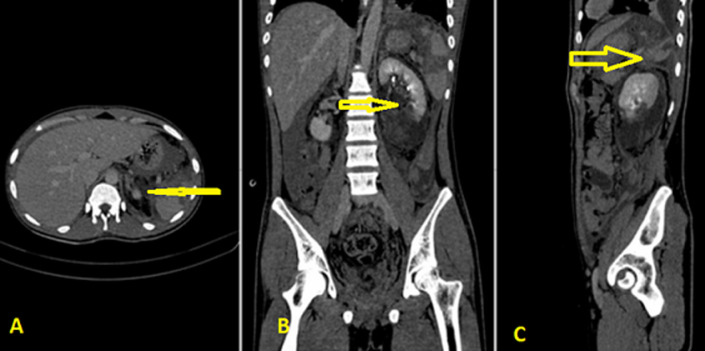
computed tomography scan showing lacerated and devascularized spleen and transected left kidney (arrows)
